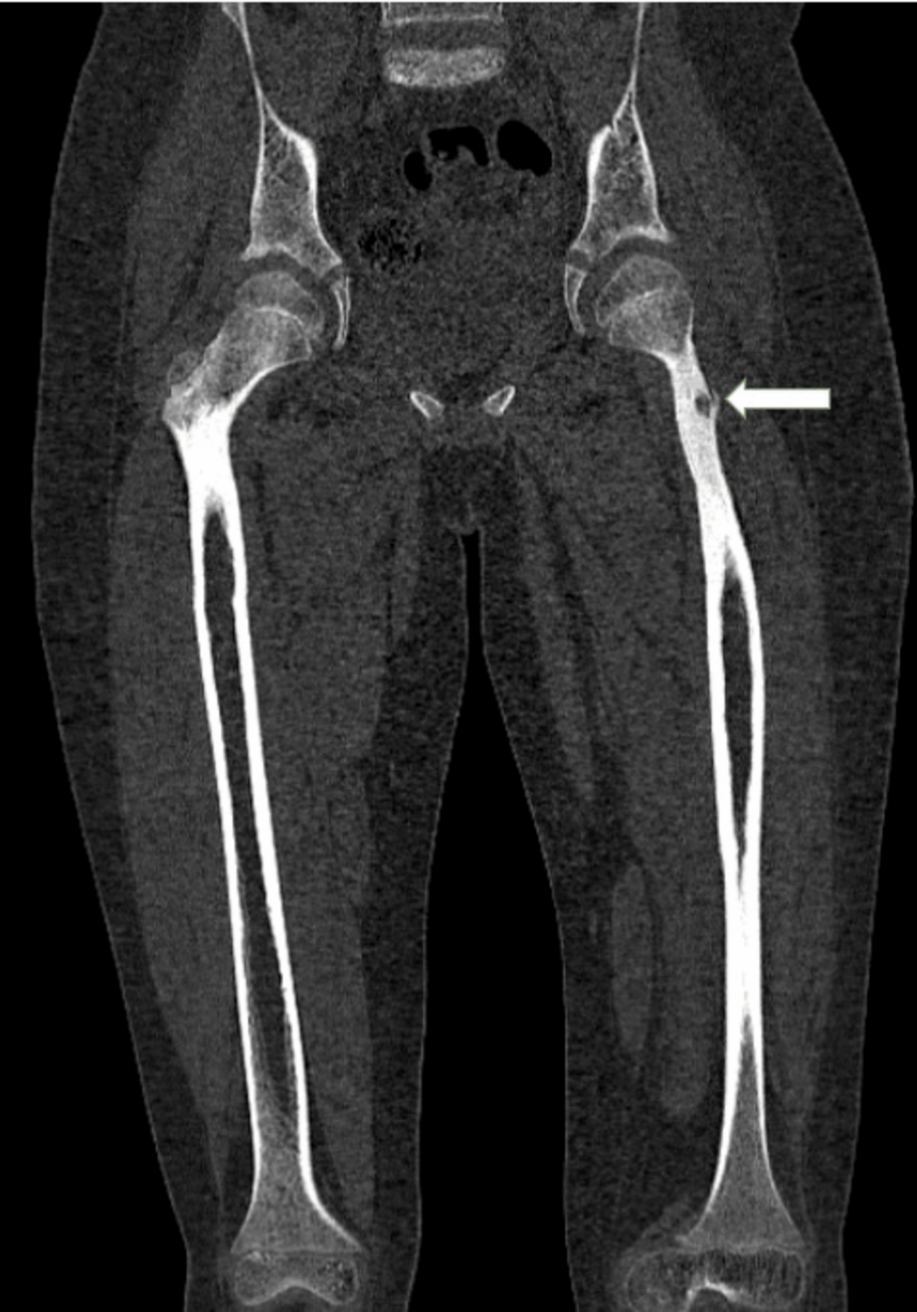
CT post radio frequency ablation revealing evidence of a well-defined lytic lesion with adjacent cortical sclerosis and anterior cortical break in the neck of the left femur showing punctate calcific focus within (white arrow)
